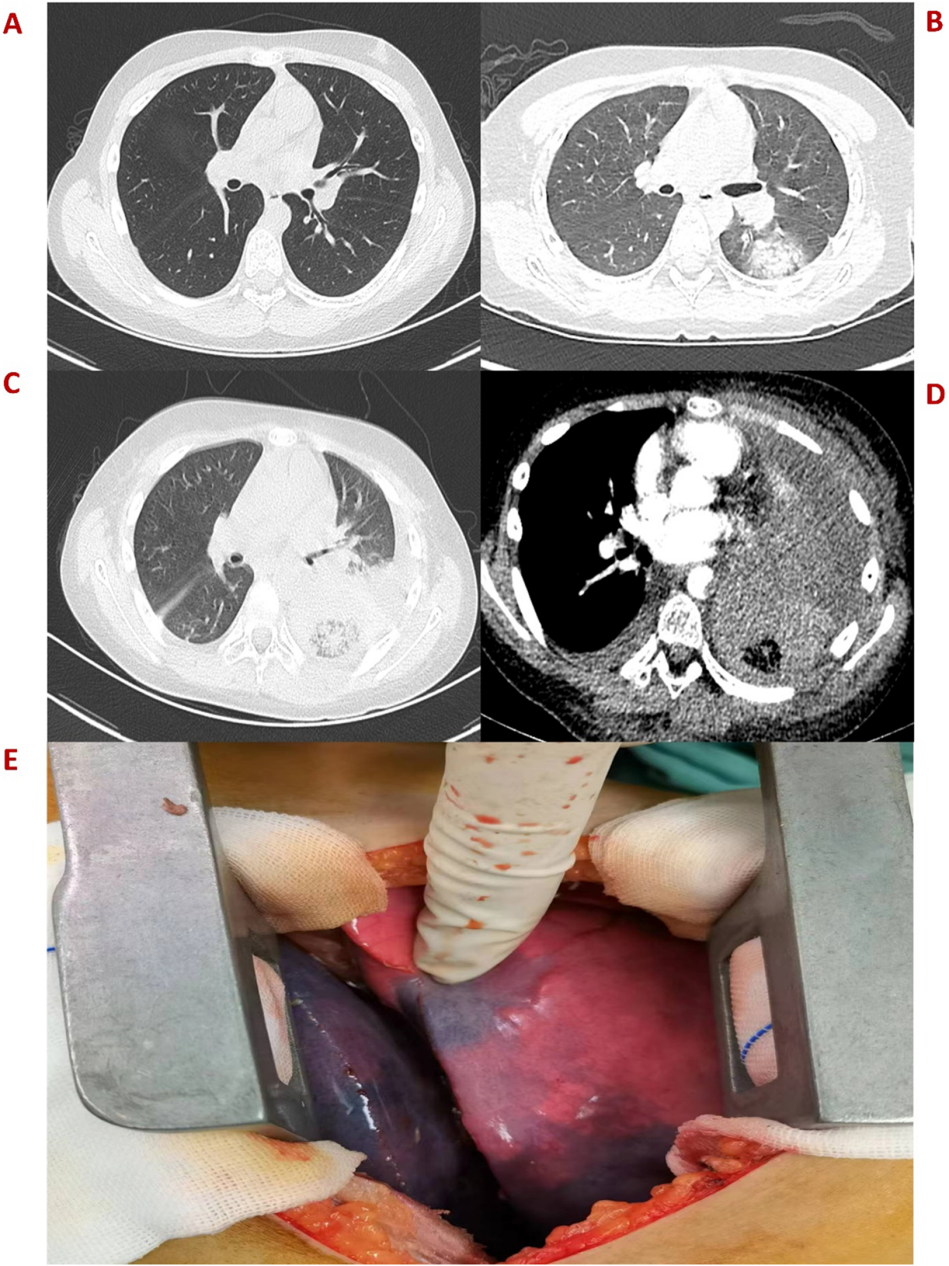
(A) Plain CT scan image of the left lung on day 2. (B) An emergency CT scan image obtained after 12 days indicates patchy ground-glass shadows in the dorsal segment of the left lower lobe. (C) An enhanced CT image obtained after 22 days shows a reverse halo sign in the left lung. (D) Enhanced CT image obtained on day 30 presents the left lung infarction. (E) Surgical photos of lesions showing the left lower lobe infarction.
